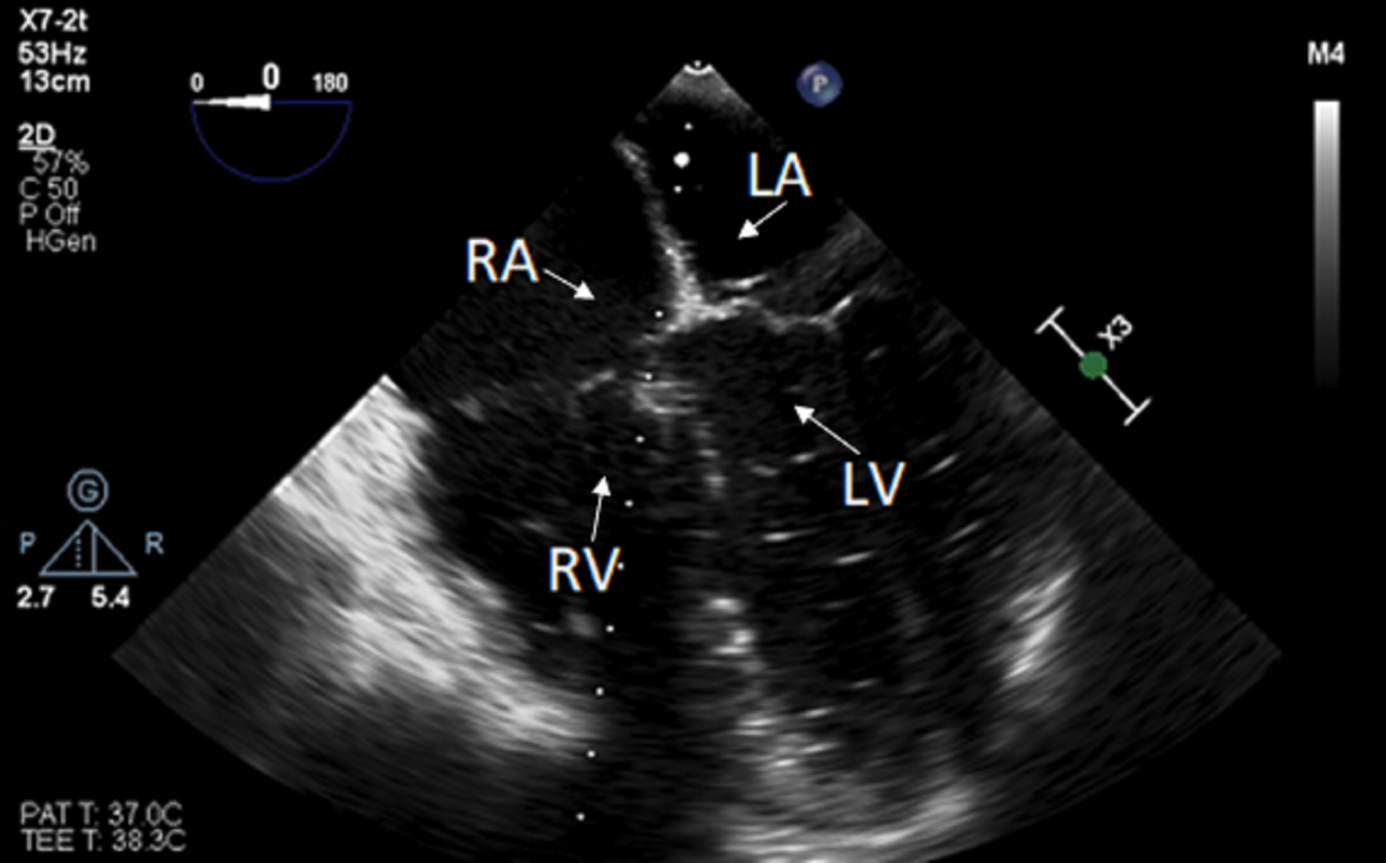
Intraoperative transesophageal echocardiogram, mid-esophageal 4-chamber view showing entrainment of air into the left-sided chambers despite a lack of intracardiac shunts identified. RA: Right Atrium; LA: Left Atrium; RV: Right Ventricle; LV: Left Ventricle.
